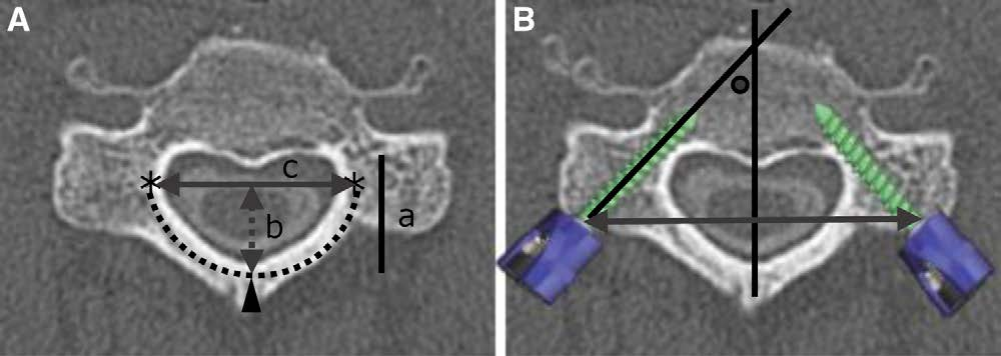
Preoperative measurements using CT images. (A and B) Transversal images at the level of C3 pedicle. (A) dotted line: Outer edge of the cortical bone, which is the wall forming the posterior part of the spinal canal. A, *: Left and right LE points. A, ▲: The most posterior point of the cortical bone of the spinal canal. Line a: Distance from the posterior wall of the vertebral body to the most posterior point of the cortical bone of the spinal canal. Double-arrow dotted line b: Distance between the line connecting the left and right LE points and the most posterior point of the cortical bone of the spinal canal. Double-arrow line c: Distance between the left and right LE points. B, ●: Insertion angle of a pedicle screw. B, double-arrow line: Width of the insertion point of pedicle screws.
